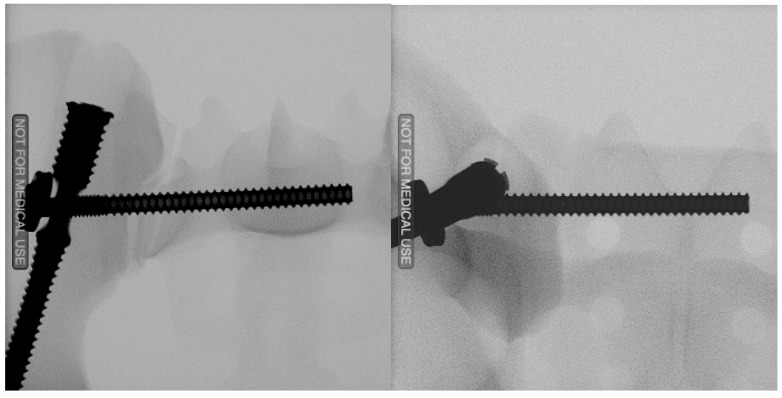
Radiograph of the TriFix fixation in two planes.
